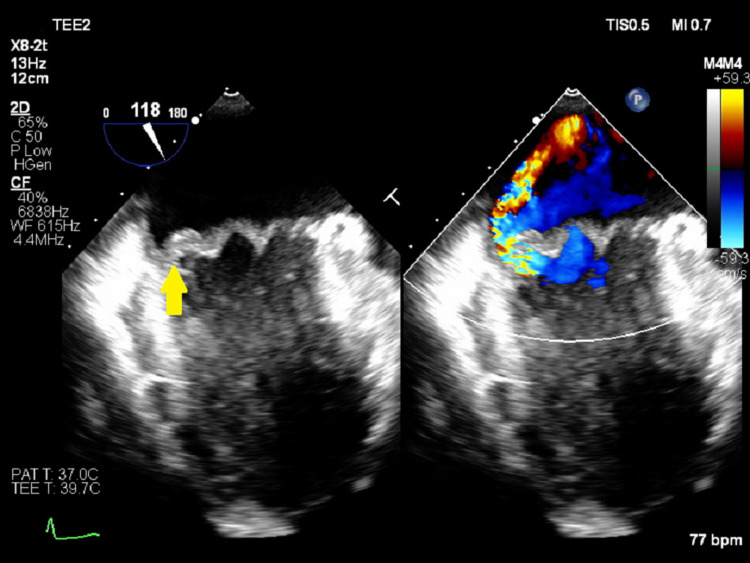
Transesophageal echocardiography findings revealed mitral regurgitation with vegetations (arrow), which had not been observed before.
